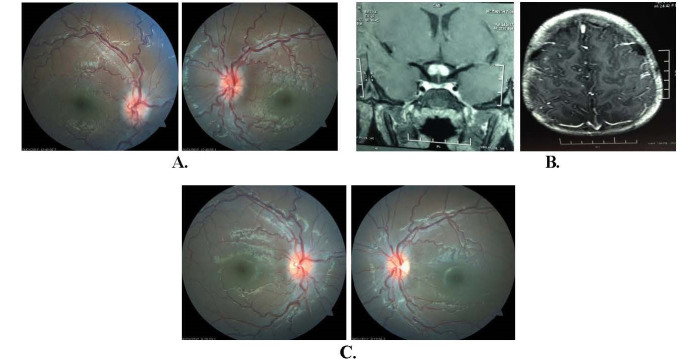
Fundus photography and radiological imaging of case 2 A. Fundus Photograph of Case 2 showing bilateral disc oedema with arteriolar attenuation and venous dilatation on Day 1; B. Contrast enhanced MRI of brain showing multiple enhancing nodular lesions in bilateral cerebral hemispheres at grey white matter interface suggestive of inflammatory tuberculomas; C. Fundus photograph showing improvement in bilateral optic neuritis on Day 3 of treatment with parenteral methylprednisolone under cover of anti-tubercular treatment
